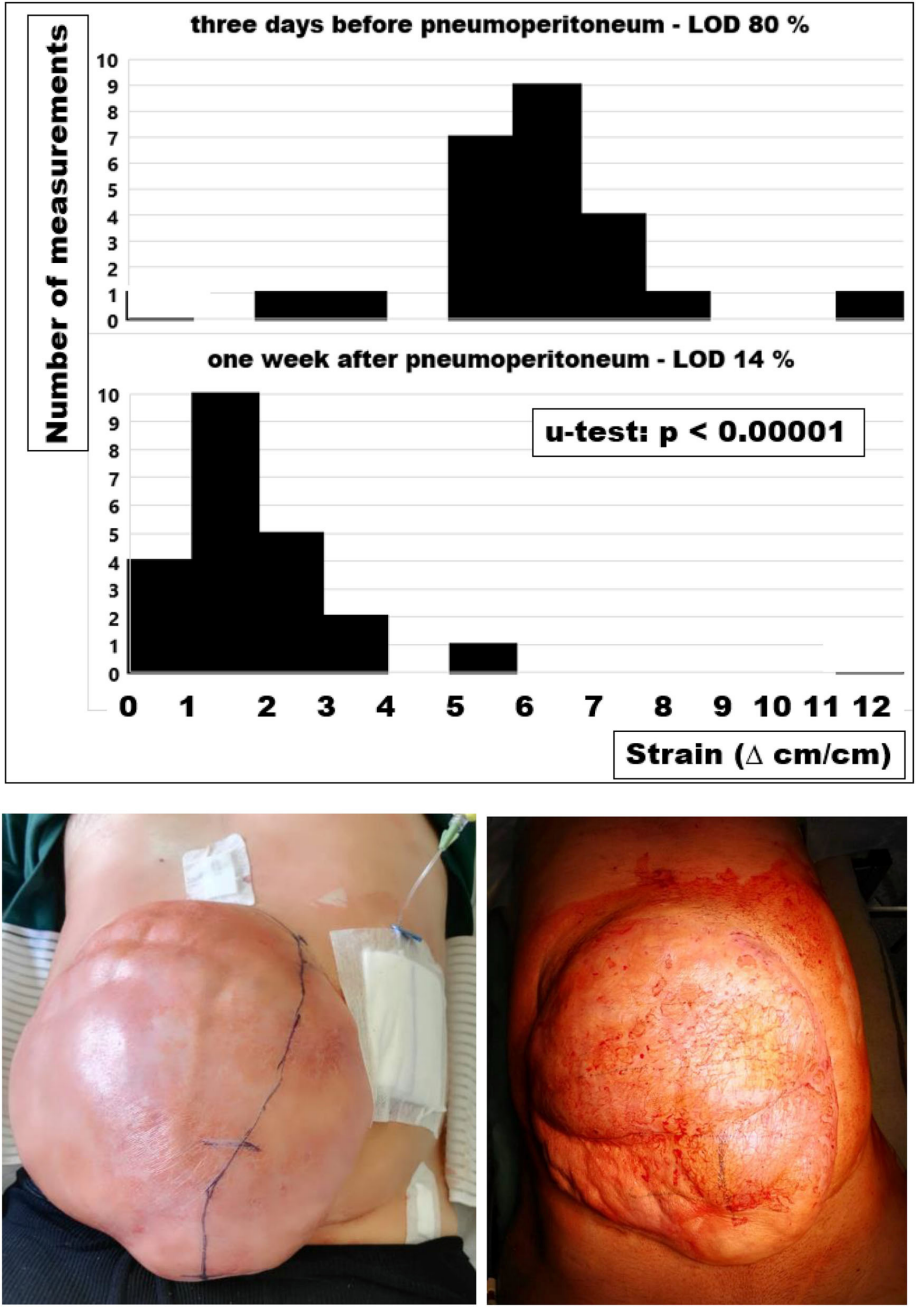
Strain assessed as the regional shift of tissue taken from computed tomography of the abdomen at rest and during a Valsalva maneuver before and after a pneumoperitoneum (top and middle) and photos taken during the first filling of the pneumoperitoneum (bottom left) and before reconstruction of the abdominal wall (bottom right). The pneumoperitoneum catheter was inserted under laparoscopic control and the incision line was free of adhesions marked under direct vision (bottom left). The tissue distension for three weeks shifts the strain distribution to the left indicating a net gain of peritoneal volume. The strain changes with progressive pneumoperitoneum markedly reduce the loss of domain, ease the shift of the viscera into the peritoneal cavity and decrease the tension on the newly formed abdominal wall after the reconstruction (*u*-test: *p* < 0.00001).
